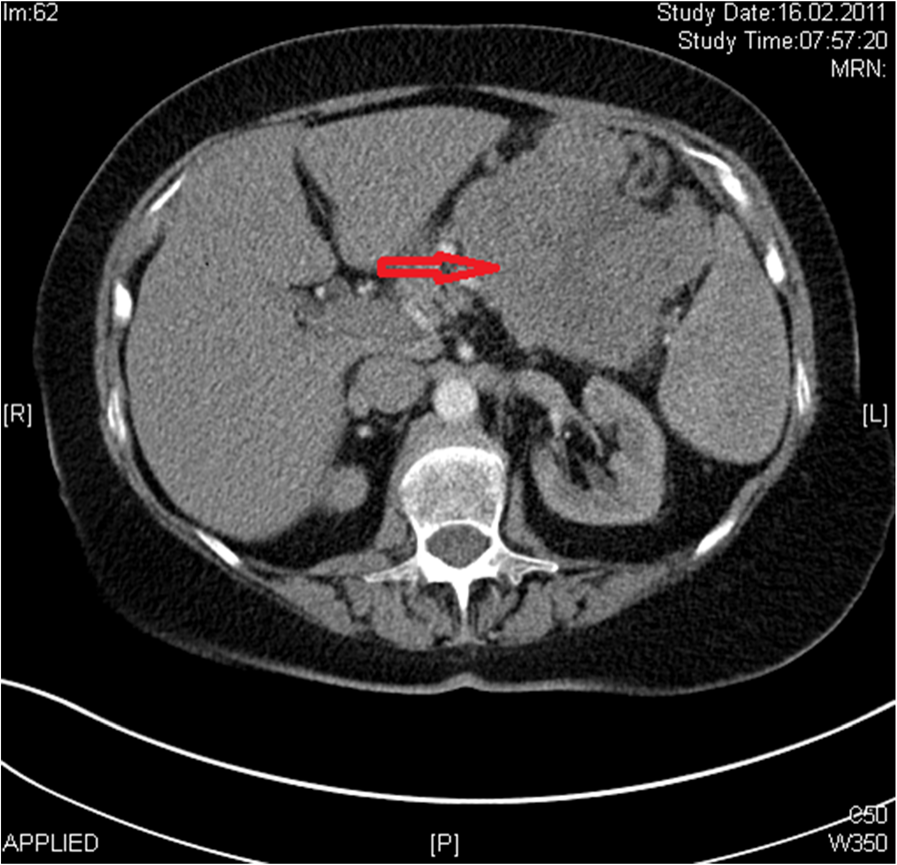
CT scan of the abdomen demonstrating a large mass in relation to the pancreatic tail.
